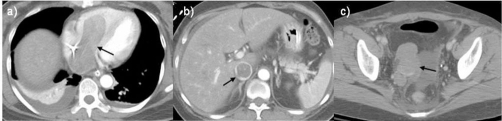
Computed Tomography images. CT of (a) the chest, (b) abdomen and (c) pelvis (soft-tissue gating). (a) A large hypodense filling defect is seen, 3.1 cm in diameter, traversing the right atrium to the right ventricle (arrow) with bilateral small pleural effusions and atelectasis. There was no evidence of a pulmonary embolism (images not shown); (b) Distension of the inferior vena cava (IVC) with the same tissue density thrombus (arrow) as was seen in (a) and with evidence of some linear enhancement, difficult to appreciate here, suggestive of a tumor thrombus; (c) A 6.2 x 3.9 cm lobulated pelvic mass (arrow) which was inseparable from the internal iliac vein.
